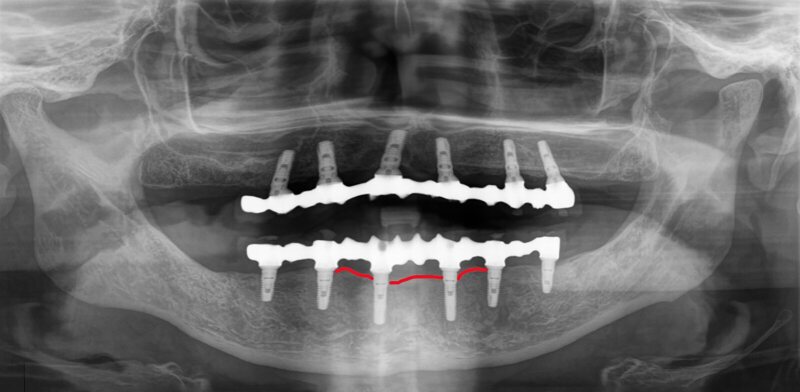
Radiographic follow-up with marked bone height from 01/2019 (a) and 09/2022 (b). The bone recession is clearly visible here.
